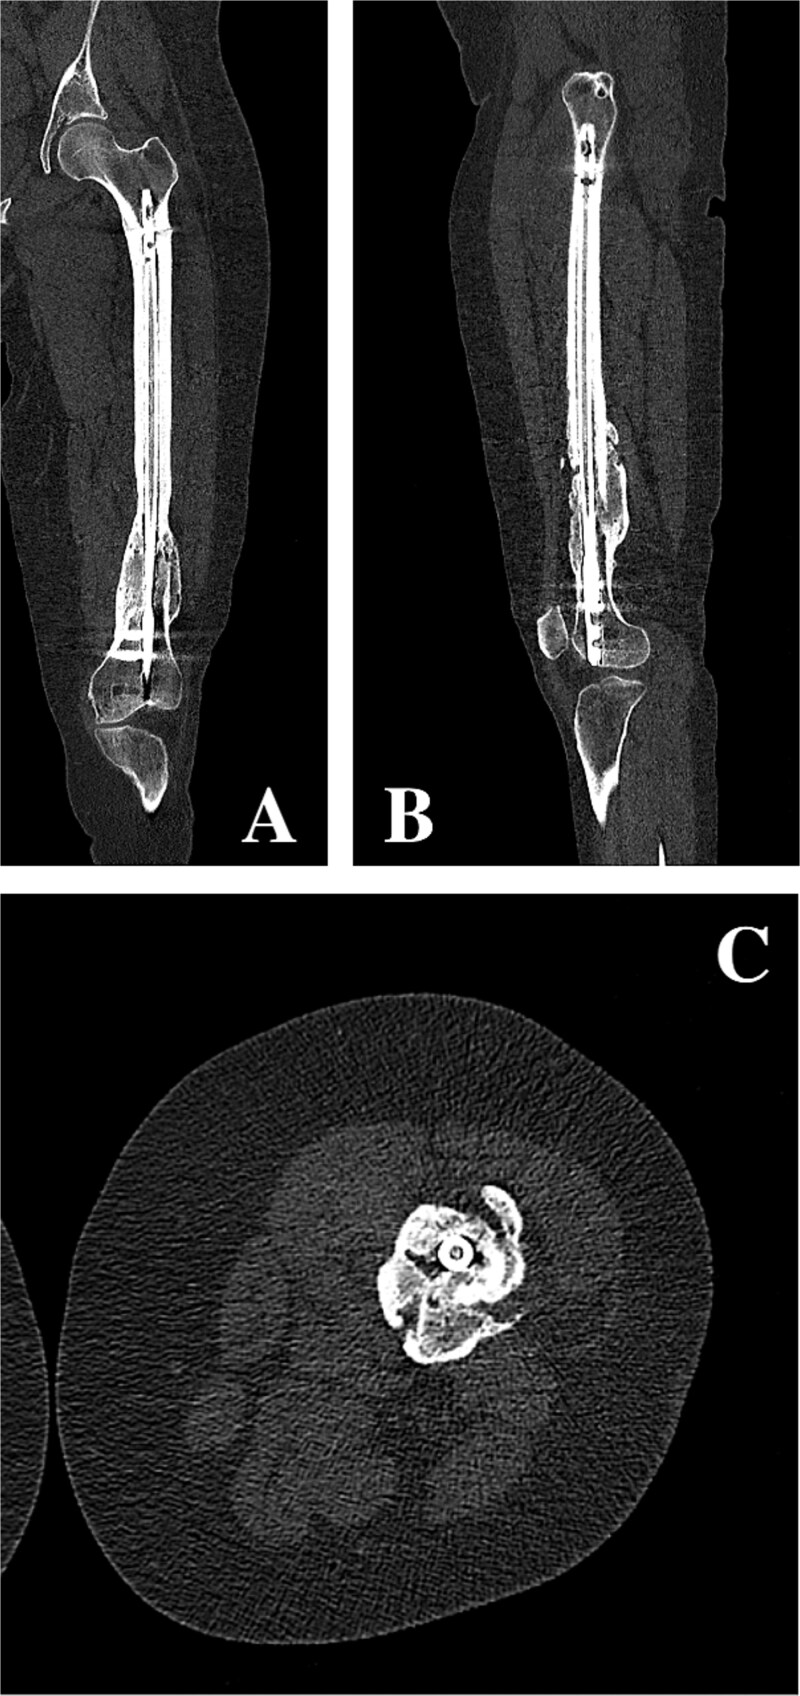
(A, B) Coronal and sagittal CT reconstructions of the left femur demonstrating an intramedullary femoral nail with extensive hypertrophic callus formation at the site of a chronic distal femoral malunion, along with an oblique proximal periprosthetic fracture adjacent to the proximal locking screw. (C) Axial CT image demonstrating hypertrophic callus consistent with a distal femoral malunion surrounding an intramedullary femoral nail.
